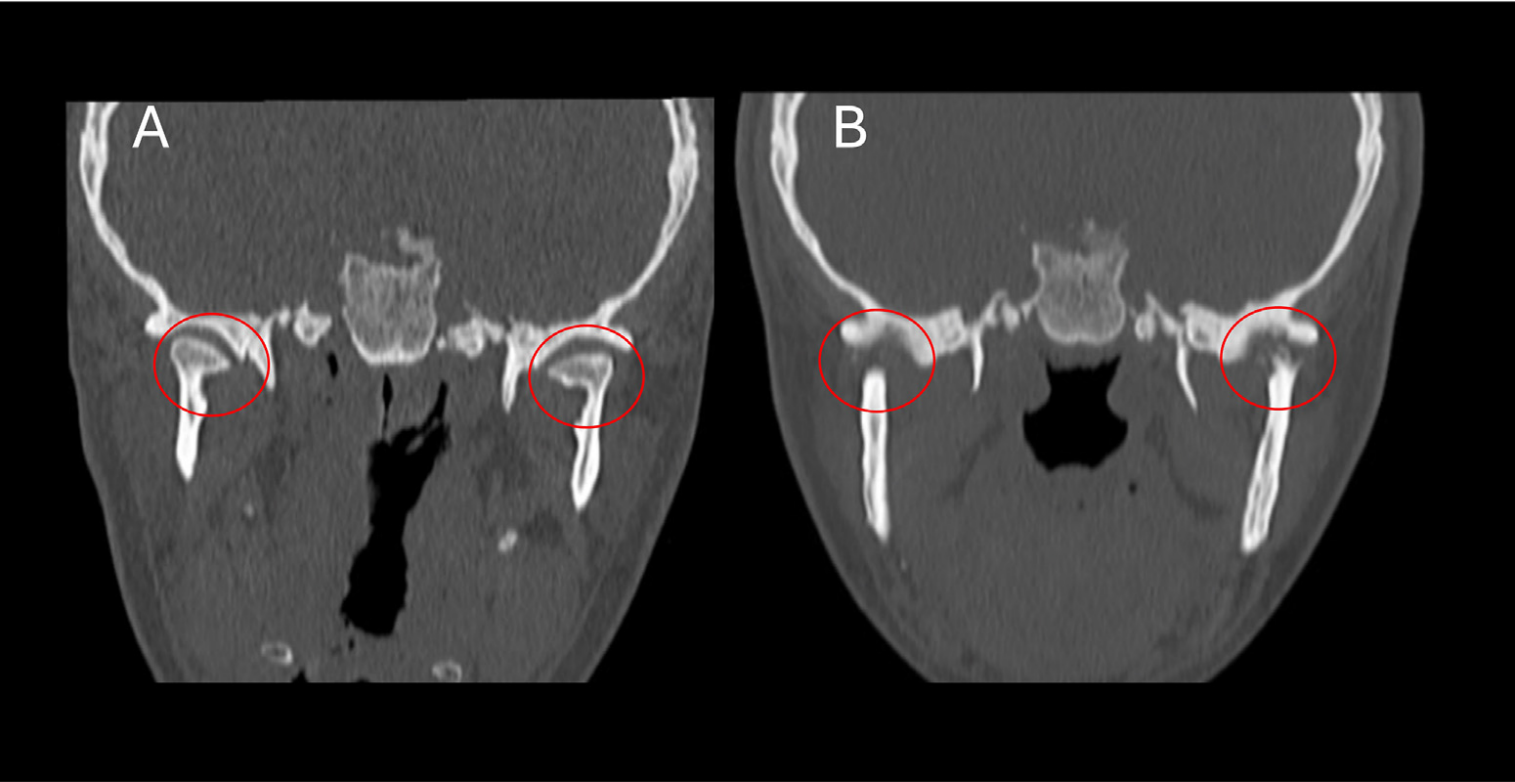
CT Image of TMJ. Coronal view (A) Before orthognathic surgery. Normal condylar process and (B) 2 years after procedure incredible loss of bone volume in the heads of mandible and flattening of mandibular fossa.
